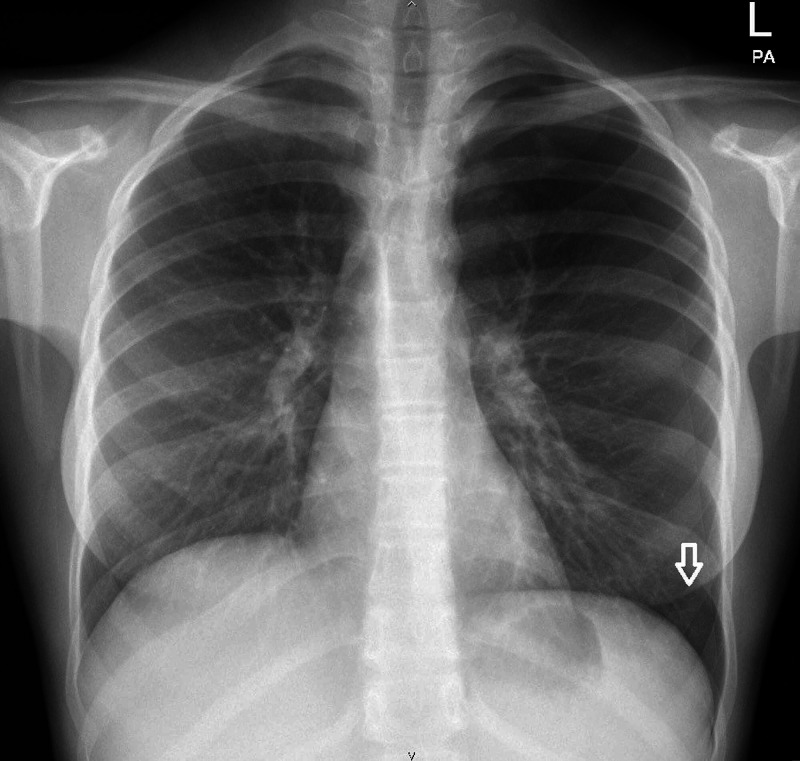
Chest X-ray showing left spontaneous pneumothorax.
